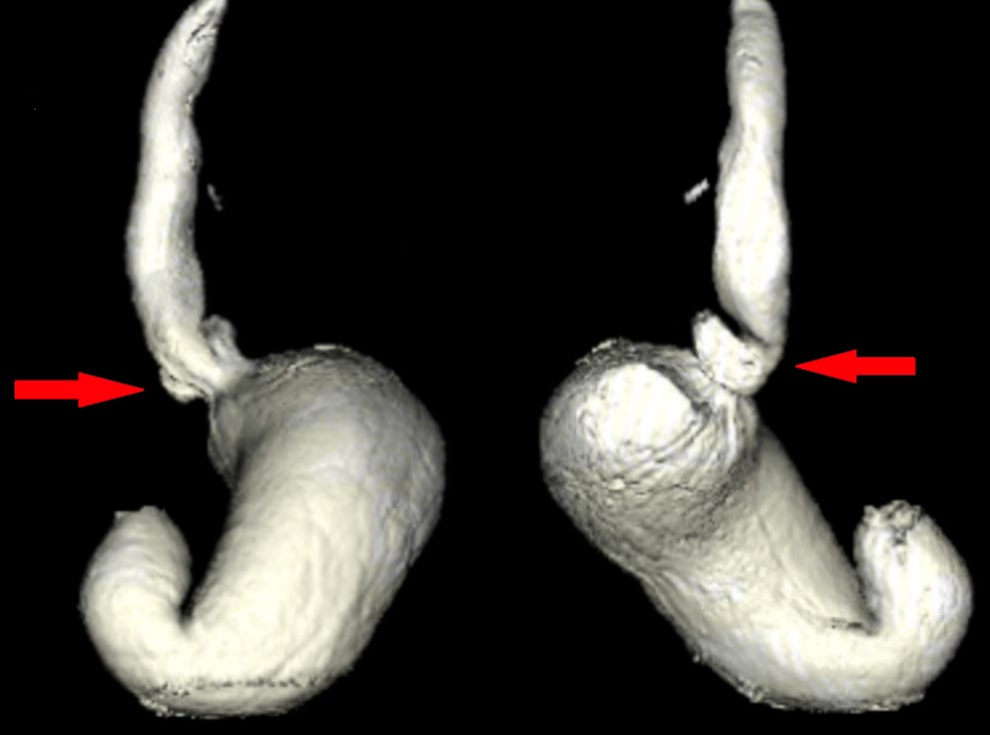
Preoperative investigation of patient #5 (77-year-old male with reflux symptoms) with 3D volumetric rendering of a Fizz-CT demonstrating a primary hiatus hernia (red arrow)
